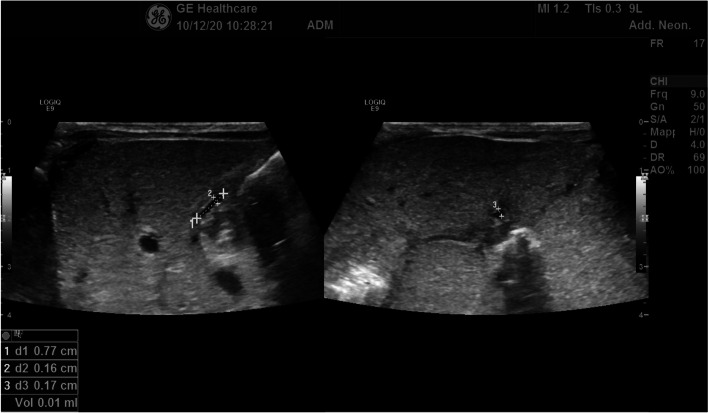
Abdominal ultrasound at 35 days of extrauterine life of the twin with jaundice: in the liver, a small and atretic gallbladder, not expanding after 3 h fasting, confirmed suspicion of biliary atresia
